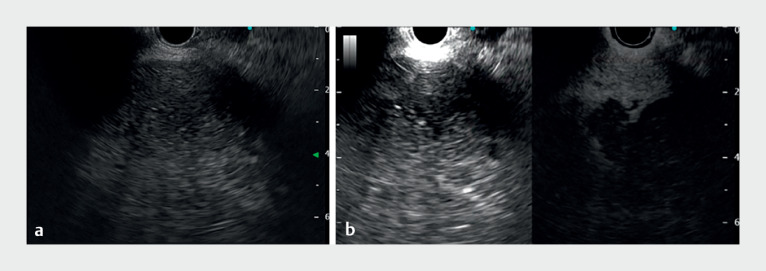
Endoscopic ultrasound (EUS) images using:
